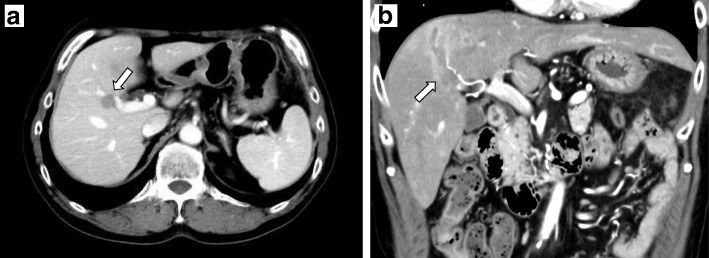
Enhanced abdominal computed tomography. a Imaging showed portal vein thrombosis in P8 (white arrow). b The tumor thrombosis was extended from P8 to the root of P5, and slight inflammation surrounding the portal vein was detected (white arrow)
